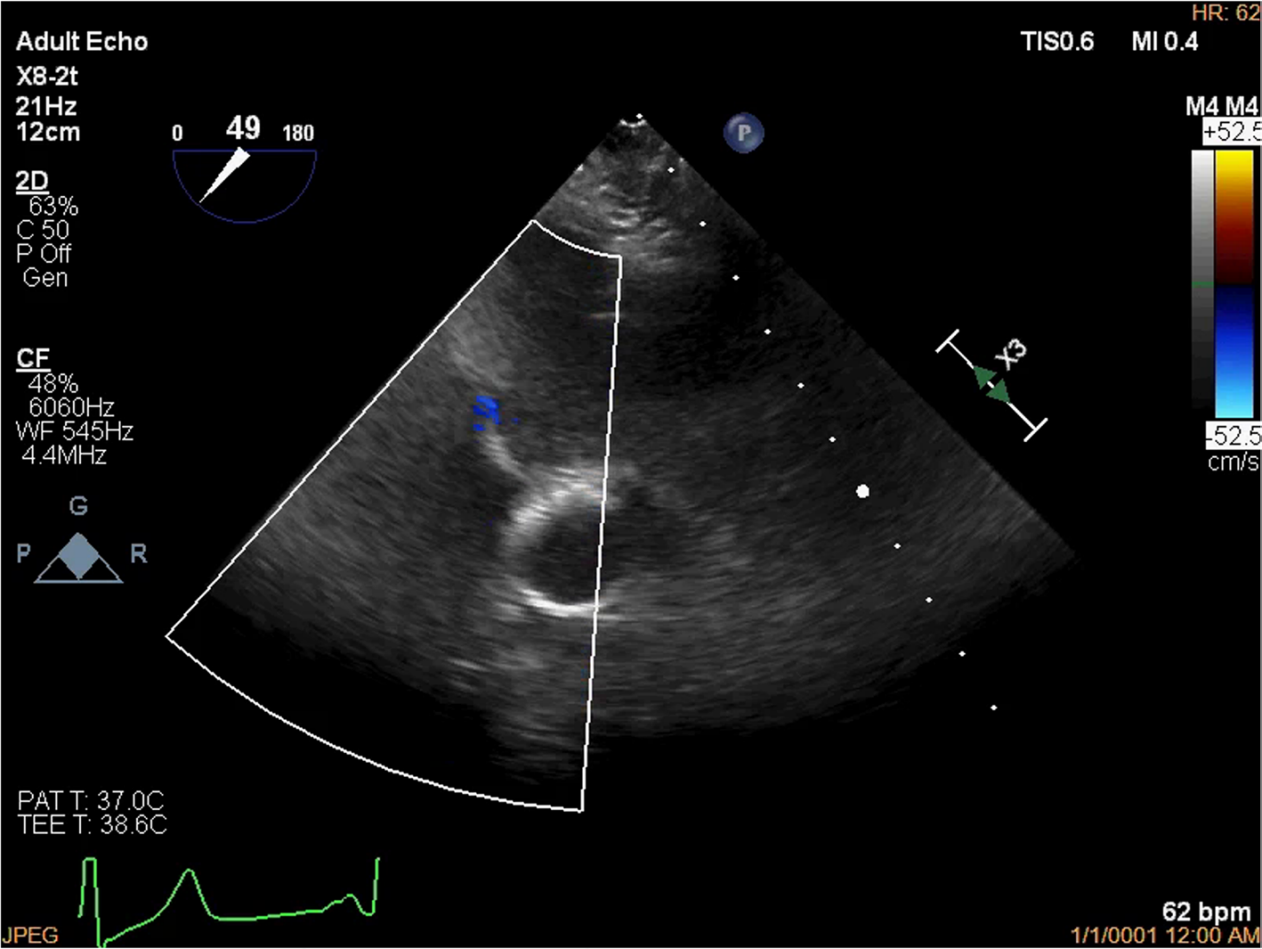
Transesophageal echocardiography image after induction of anesthesia. This is the only transesophageal echocardiography image we recorded after induction of anesthesia. Because of poor image quality due to loose contact of the TEE probe to the reconstructed esophagus mucosa, the aortic valve was barely visible
